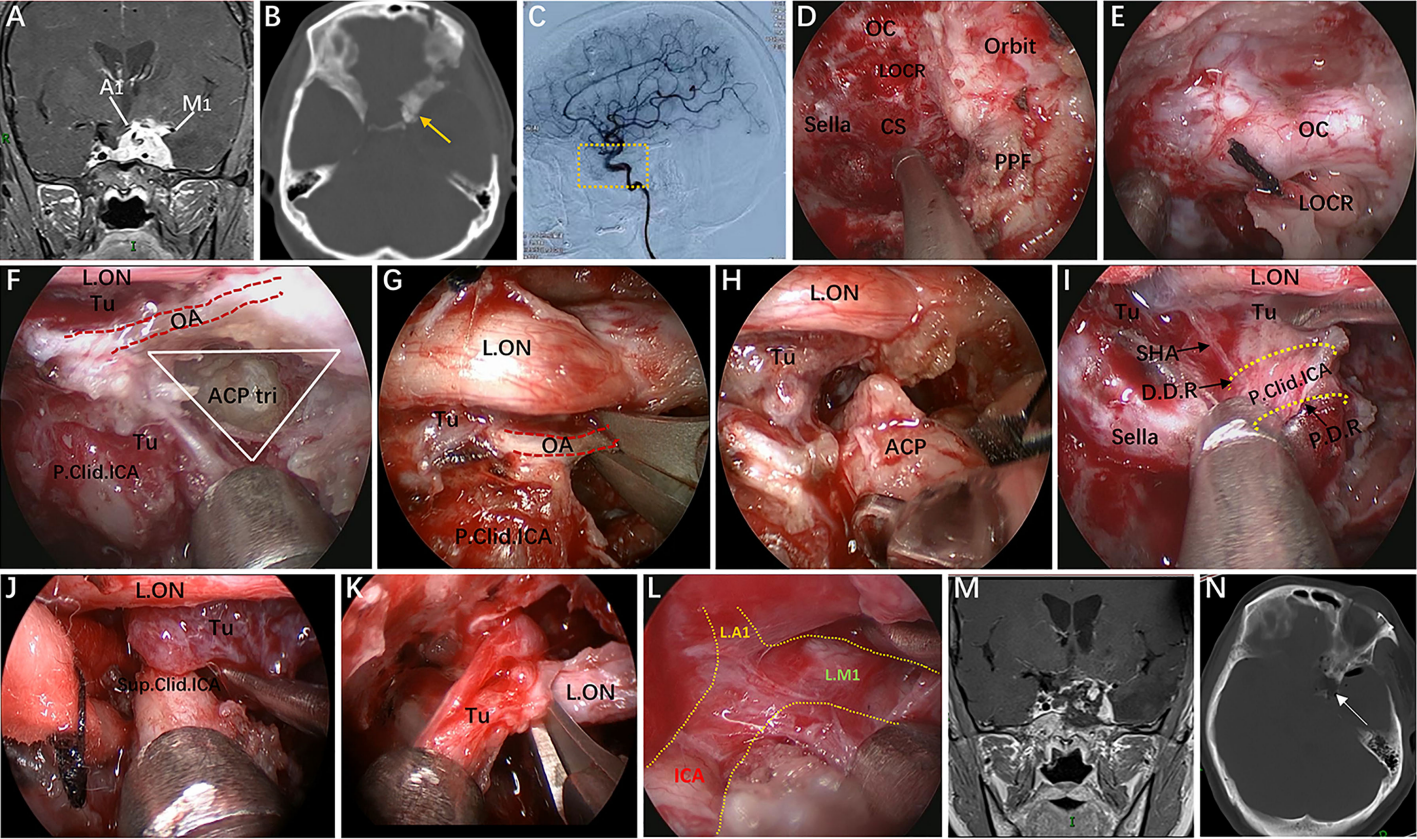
Case 4, a 37-year-old woman, presented with progressive visual loss in the left eye for 2 years. (A) Preoperative coronal Gd-enhanced MRI showing a left recurrent ACP meningioma involving the right cavernous sinus and encasement of the ICA and its bifurcation. (B) Preoperative CT scans. The yellow arrow indicates the hyperplastic ACP. (C) Preoperative lateral view of left ICA injection of digital subtraction angiography (DSA) showing that the ophthalmic artery was not visible. (D–L) Intraoperative images. (D) Endoscopic view of important bony landmarks after the initial transpterygoid approach. (E) Exposure of the thickened OC and drilling of its roof and anterior walls. (F) Endoscopic exposure of the ACP triangle. The red dotted line indicates the course of the imaginary occluded OA. (G) Sacrifice of the OA surrounded by tumors and involving the dura. (H) Removal of the hyperplastic ACP. (I–K) Resection of the tumor on the medial and lateral sides of the supraclinoidal ICA through the combined supraoptic and infraoptic approaches. (L) Endoscopic exposure of the bifurcation of the ICA covered by arachnoid membrane. (M) Corresponding postoperative coronal MRI showing subtotal tumor resection, with part of the tumor remaining in the lateral wall of the left CS. (N) Postoperative CT scan showing ACP resection. The white arrow represents the removed ACP. Some panels (9A, B, M, N) of the figure have been published in *Journal of Neurosurgery*. Published with permission. The figure is available in color only online.
